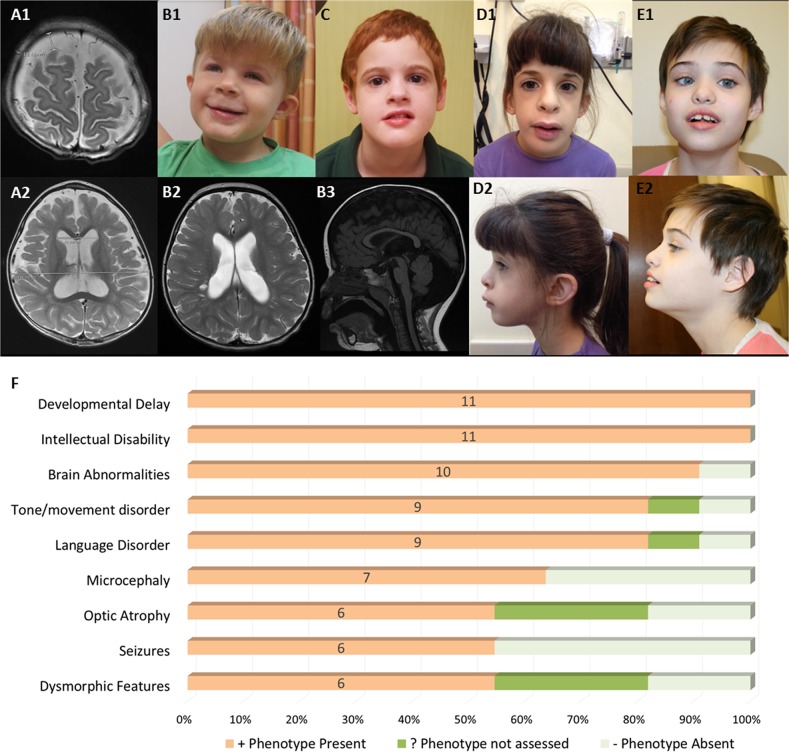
Photographs, MRI images, and phenotypes of patients with biallelic protein altering variants in *PTPN23*. Brain MRI images of Patient 1 (a1 and a2) at 14 months of age showing mild ventriculomegaly and decrease in cerebral volume. Brain MRI images of Patient 2 (b2 and b3) showing ventriculomegaly and brain volume loss. Patient brain abnormalities include ventriculomegaly, cerebral atrophy, abnormal myelination, and hypoplastic corpus callosum. Facial features observed in patients with biallelic variants in *PTPN23*: Patient 2 (b1), Patient 3 (c), Patient 5 (d1 and d2), Patient 6 (e1 and e2) included strabismus, abnormal nose and ear morphology, and broad mouth with full lips. Panel f shows the major phenotypic features identified in patients with biallelic disease causing variants in *PTPN23* including developmental delay and intellectual disability (100%), brain abnormalities (90.9%), mostly characterized by brain atrophy with cerebral volume loss, ventriculomegaly, and delayed myelination; tone or movement disorder, mainly spasticity (81.8%); language absence or delay (81.8%), microcephaly (63.6%), optic atrophy (54.5%), seizures (54.5%), and dysmorphic features (54.5%)
